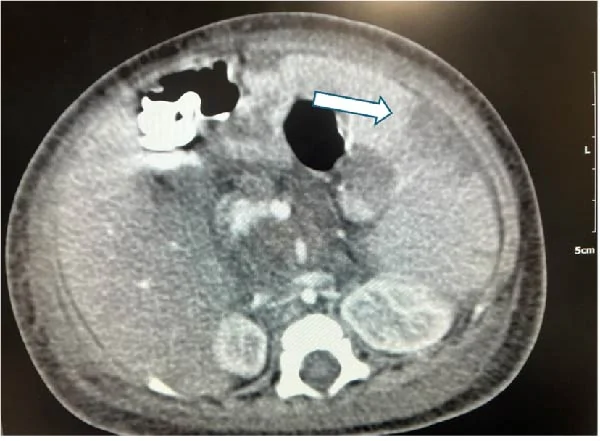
Hypodense wedge‐shaped splenic lesion on abdominal computed tomography.
